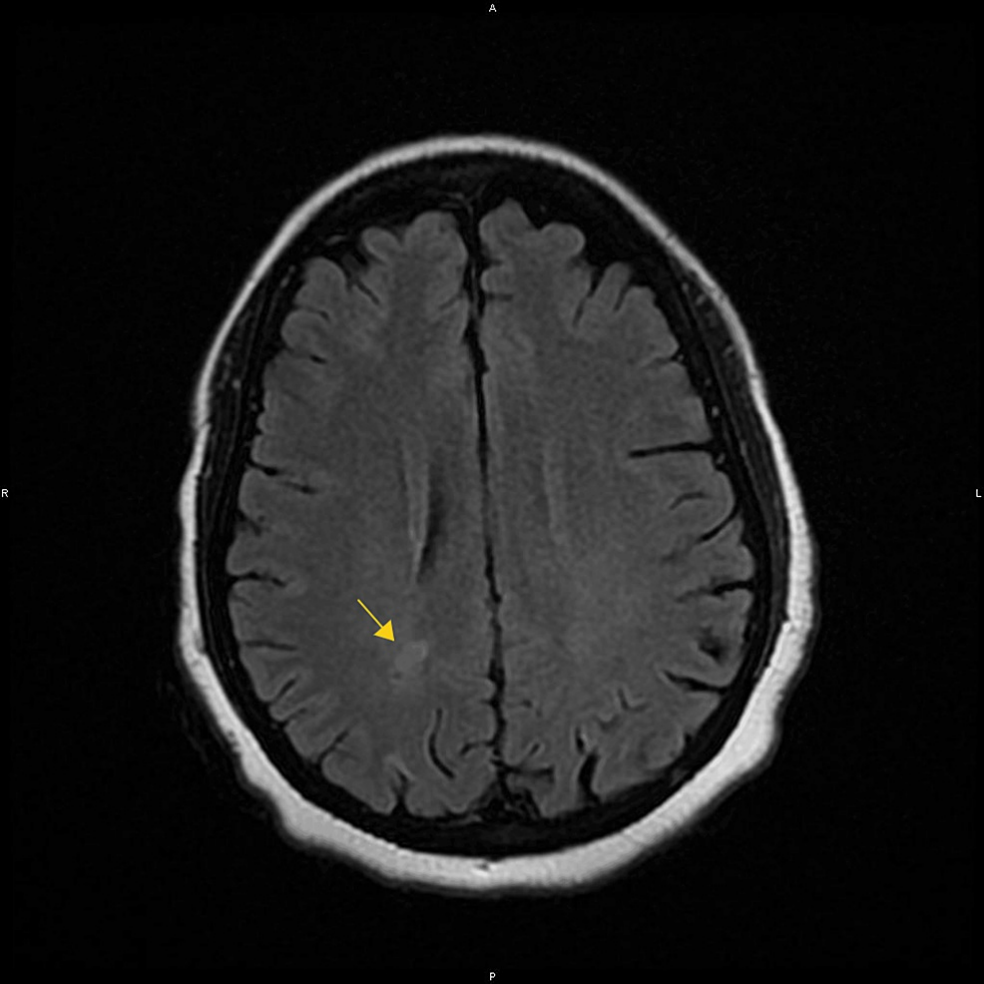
Axial FLAIR images demonstrate interval resolution of most subcortical parieto-occipital hyperintensities with minor residual Juxtacortical signal (yellow arrow) predominantly on the right parieto-occipital subcortical white matter compatible with the natural history of posterior reversible ischemic encephalopathy syndrome.
